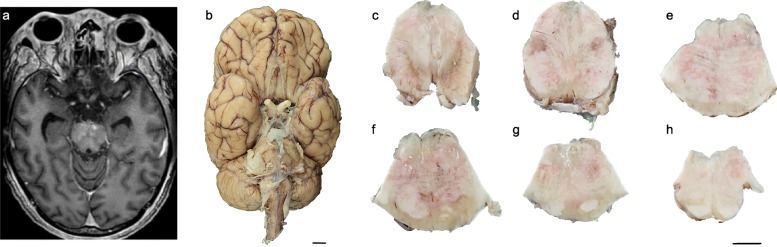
Radiologic and macroscopic autopsy findings of this patient. a T1 FLAIR weighted MRI showing multiple hyperintensities within the upper pons. b Inferior aspect of the brain demonstrating a markedly widened brainstem. c-h Transverse sections of the brainstem from rostral to caudal commencing from the caudal midbrain to the rostral medulla oblongata. Scale bars: b, 1 cm; c-h, 1 cm. The high resolution source of Figure 1 is available as Additional file1
